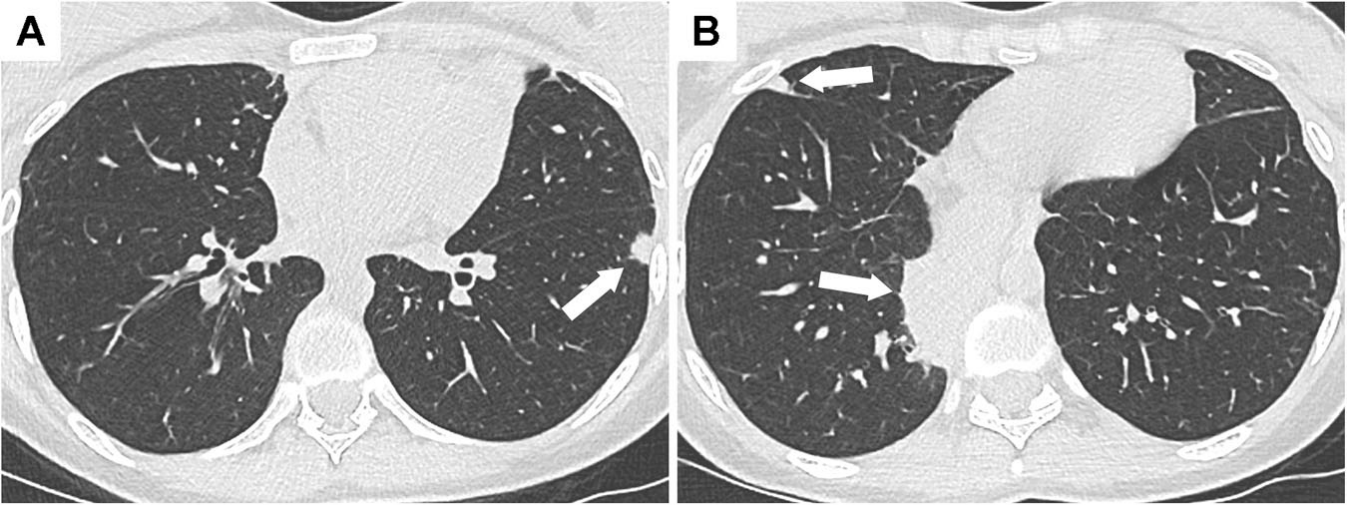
Drug-induced pneumonia in an 18-year-old patient with ulcerative colitis treated with mesalamine. CT image in the axial plane images show bilateral subpleural consolidations (arrows in A and B). CT-guided transthoracic biopsy was performed and showed pathological findings compatible with mesalamine-induced lung disease
